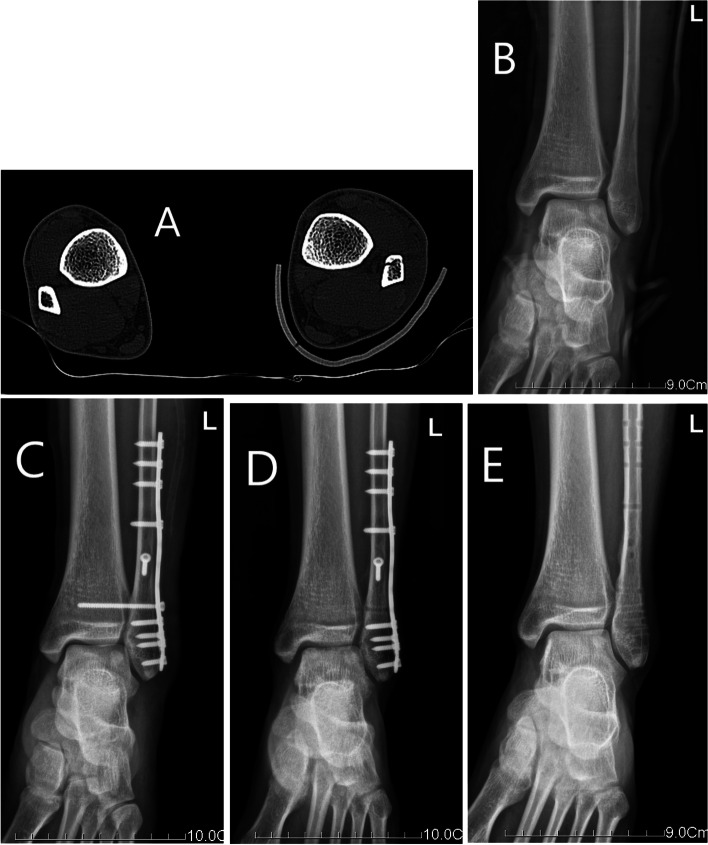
CT and X-ray images of syndesmotic screw fixation. Notes: This patient underwent the syndesmotic screw fixation. A Preoperative CT image. B Preoperative radiographs image. C Radiograph at 1 days postoperatively. D Radiograph at 3 months postoperatively. Postoperative radiographs showed ankle fracture reduction maintained well and the syndesmotic screw was removed. E Radiograph after removal of internal fixation
